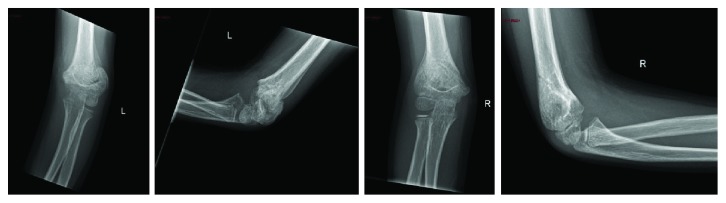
4 weeks post-op X-rays. AP and lateral views of the right and left elbows.
